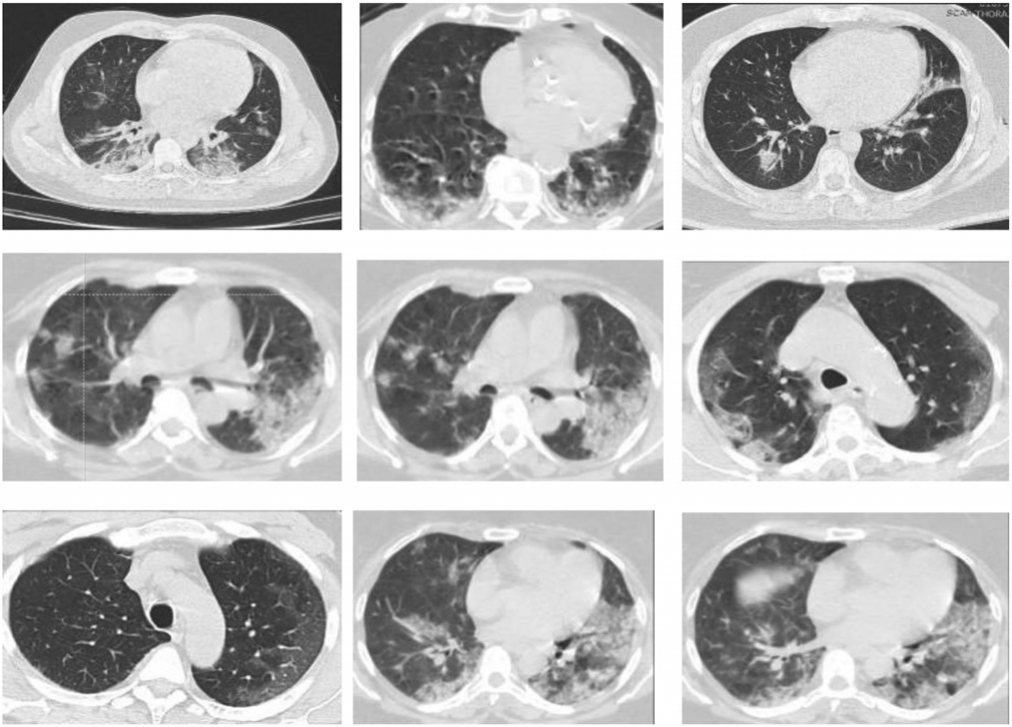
CT data images of some patients.
